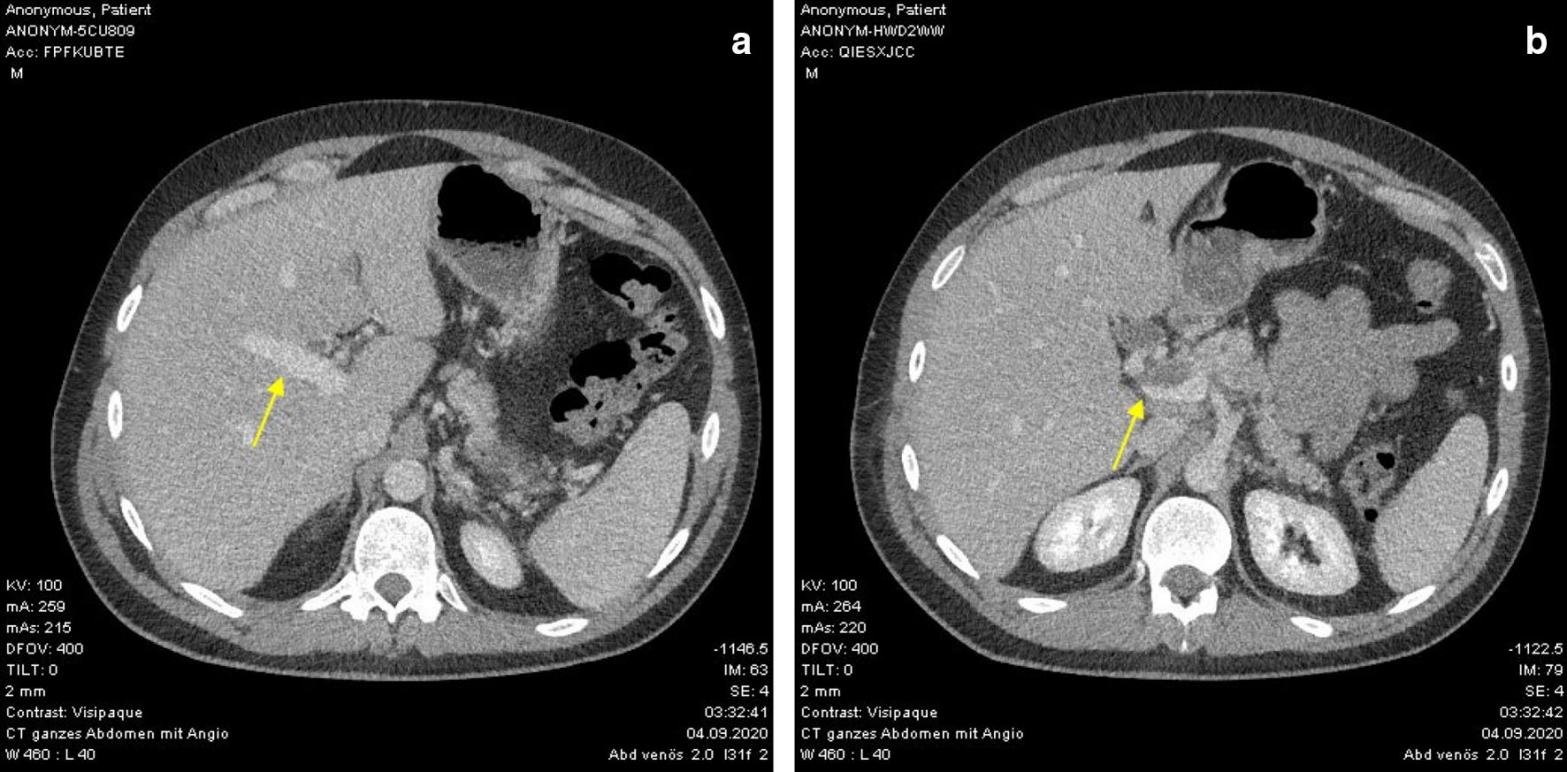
a, b CT-scan 14 days after admission with regressive portal vein thrombosis (yellow arrows)
